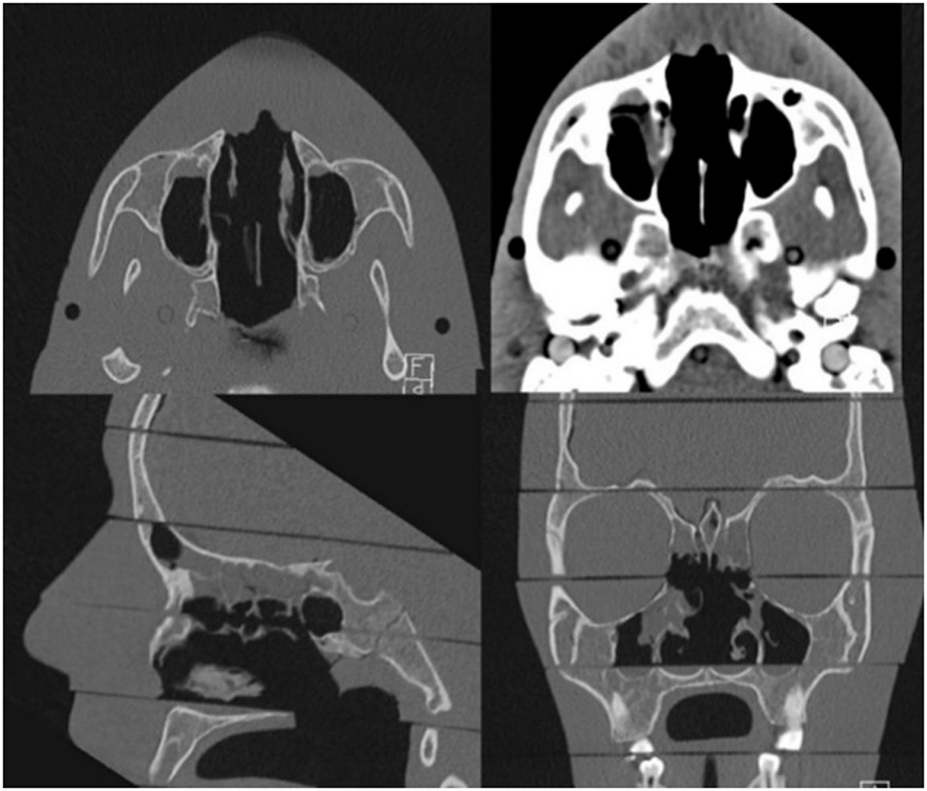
Example image panel of the online survey consisting of three CT-images in bone window in all three spatial directions and a single axial slice in a soft tissue window.
